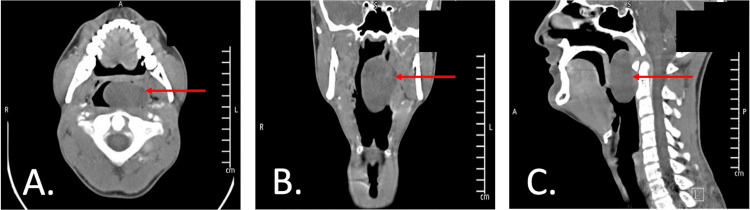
CT images (A. axial B. coronal C. sagittal) Computed tomography imaging of the neck with intravenous contrast shows a well-circumscribed low-density area of polypoid morphology arising from the left lateral pharyngeal wall, filling most of the posterior pharynx with maximum measurements of 2.6 x 3.9 x 5.3 cm.
